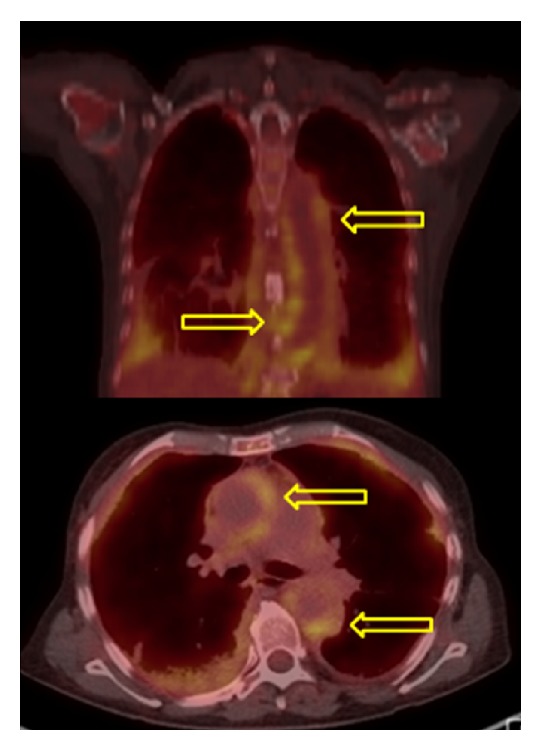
PET scan showing nodular FDG uptake in the wall of the thoracic and upper abdominal aorta and in the right atrium, suspicious for large cell arteritis.
